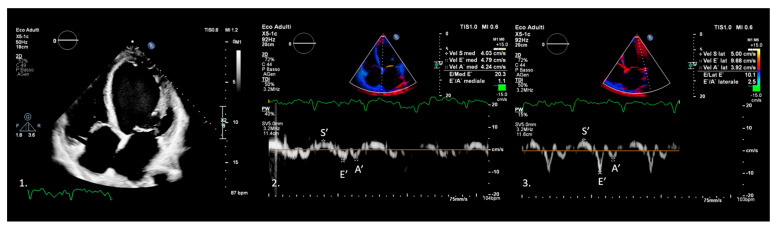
Tissue Doppler imaging (TDI) of a patient with dilated cardiomyopathy (1) showing a marked reduction in systolic and diastolic velocities at the inferior septum (2) and lateral wall (3) of the left ventricle, consistent with impaired systolic and diastolic function.
